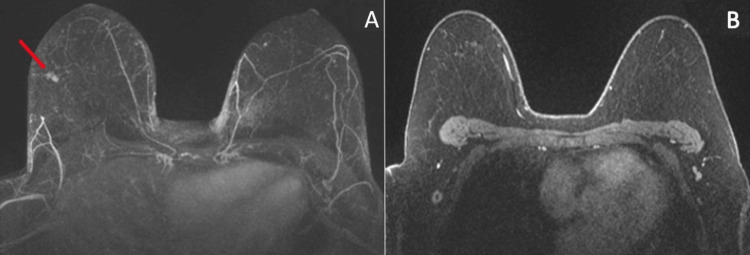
MRI findings. A. Maximum intensity projection image. B. Axial contrast-enhanced T1-weighted image. A 1 cm mass is seen at the right 8:00 position (A, red arrow). The biopsied lymph node is seen in the right axilla (B).
